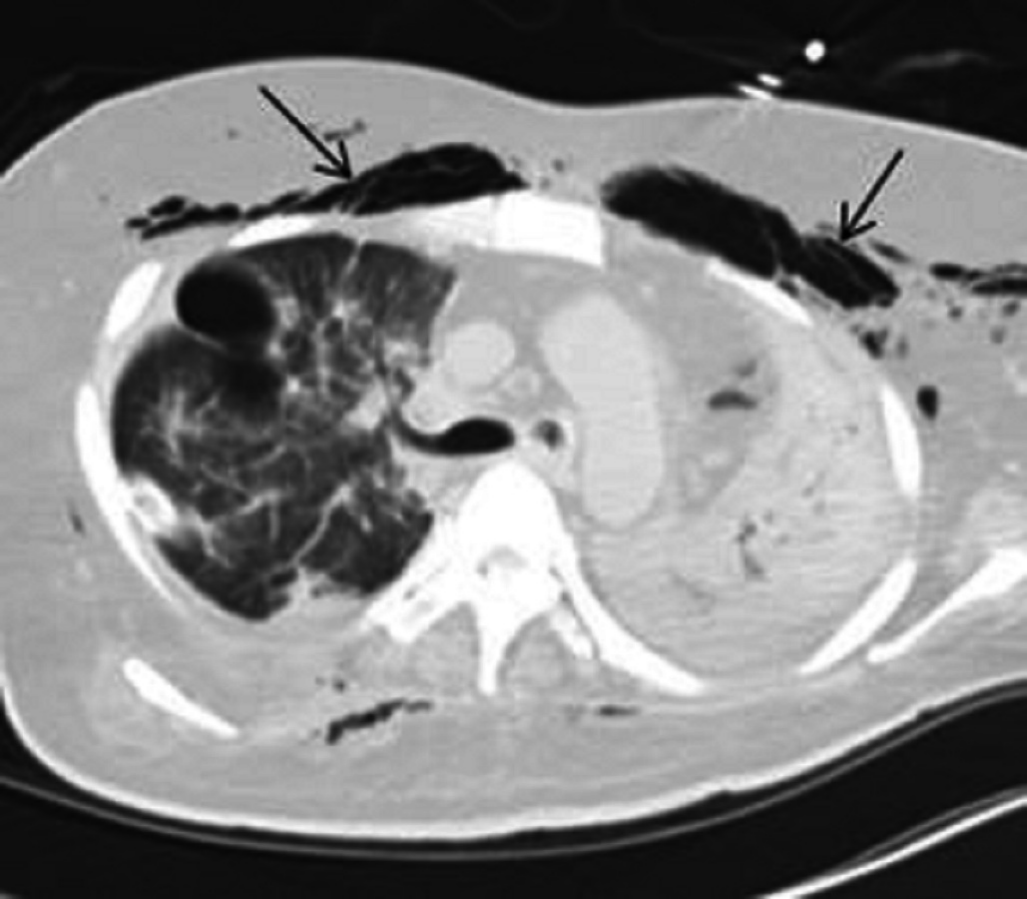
Computed tomography (CT) chest showing subcutaneous emphysema as indicated by arrows
